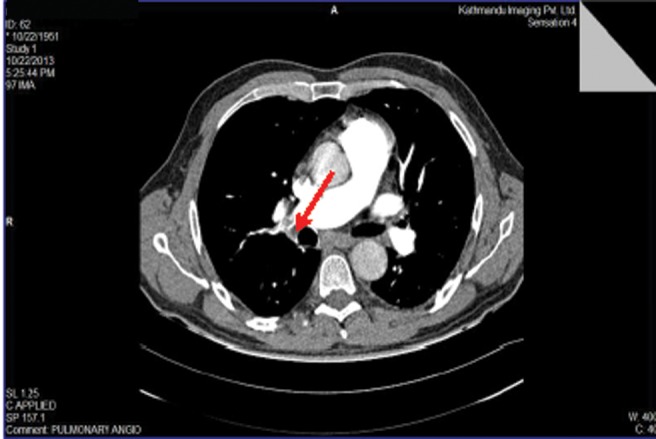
Patient 2: Axial image of CT pulmonary angiogram showing thrombi as filling defects in distal right pulmonary artery (*arrow*) with extension into right lower lobe branches.
